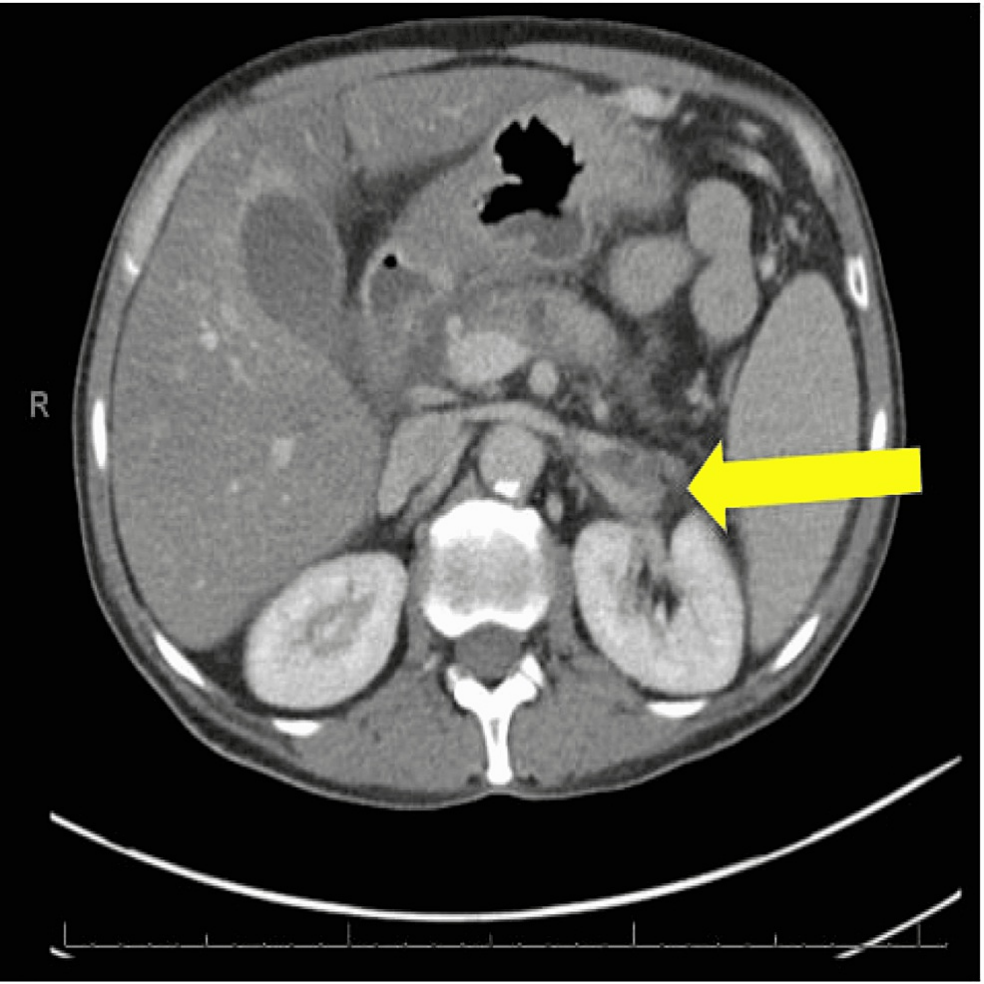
Computed tomography (cross-sectional view) with splenic vein thrombosis (yellow arrow).
